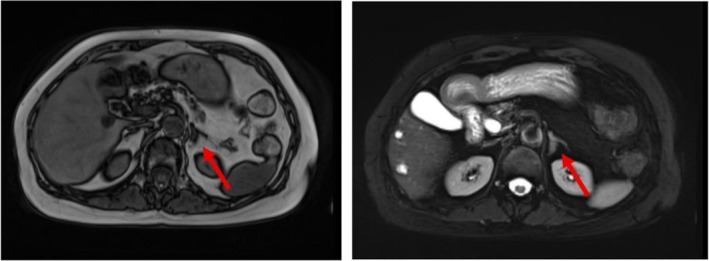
Abdominal MRI showing a left adrenal adenoma (arrow). A 7‐mm left adrenal mass was identified at the time of diagnosis of primary aldosteronism and showed no significant change in size or morphology during 13 years of follow‐up, indicating radiological stability.
